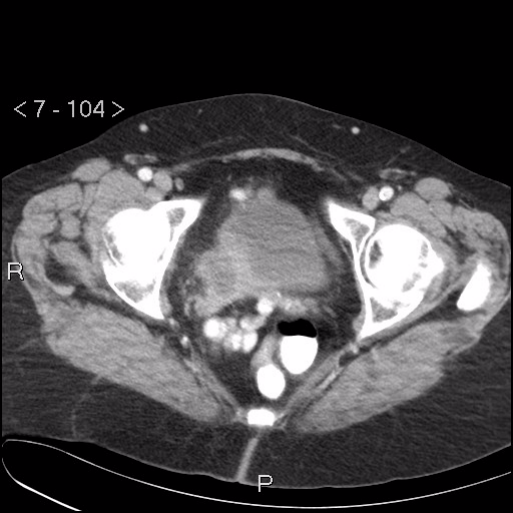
Post-contrast computed tomography scan (Siemens Positron Plus 4) showing a tumorous mass located at the right adnexal region with a broad vaginal cuff bordering a thickened bladder wall.
